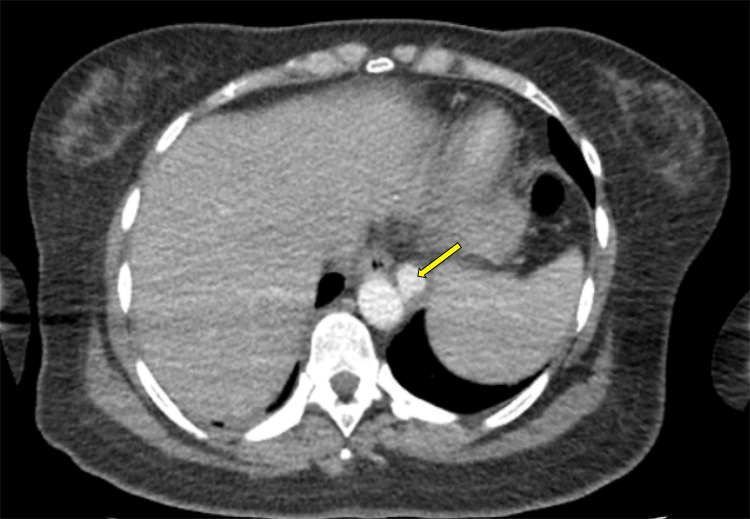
CT of the thorax showing an aneurysm in the distal thoracic aorta (arrow).
